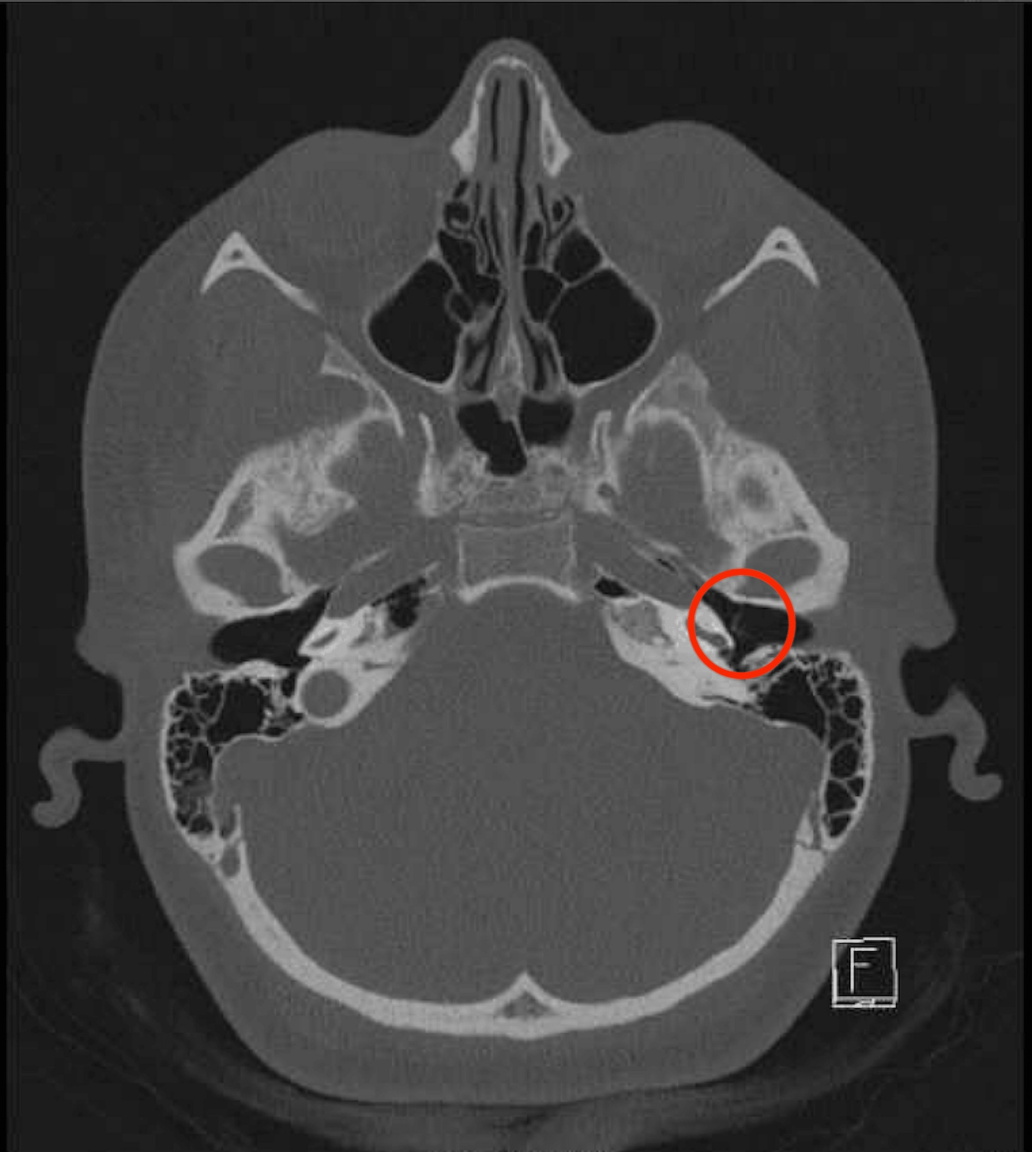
Axial view of a temporal CT scan without contrast, showing a thickened left tympanic membrane (highlighted with a red circle)
